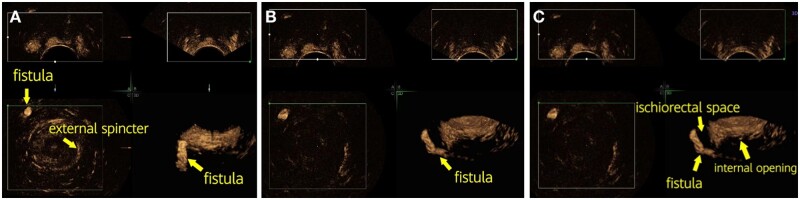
Suprasphincteric fistula and internal opening. (A) Position relationship between anal fistula and sphincter. (B) Fistula imaging under SVE 3D-TPUS. (C) Internal opening and fistula imaging under SVE 3D-TPUS. SVE 3D-TPUS, SonoVue 3D transperineal ultrasound.
